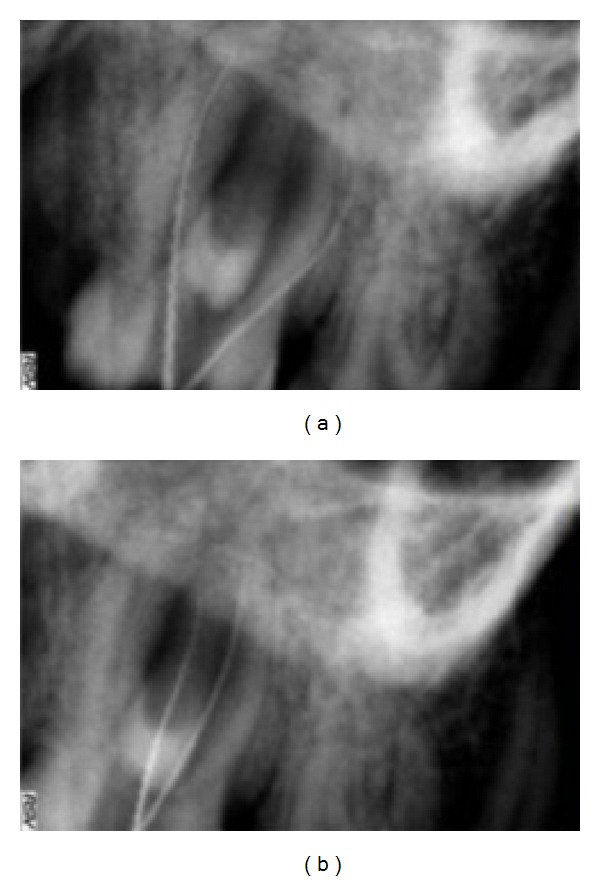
Working length radiograph of different canals.
